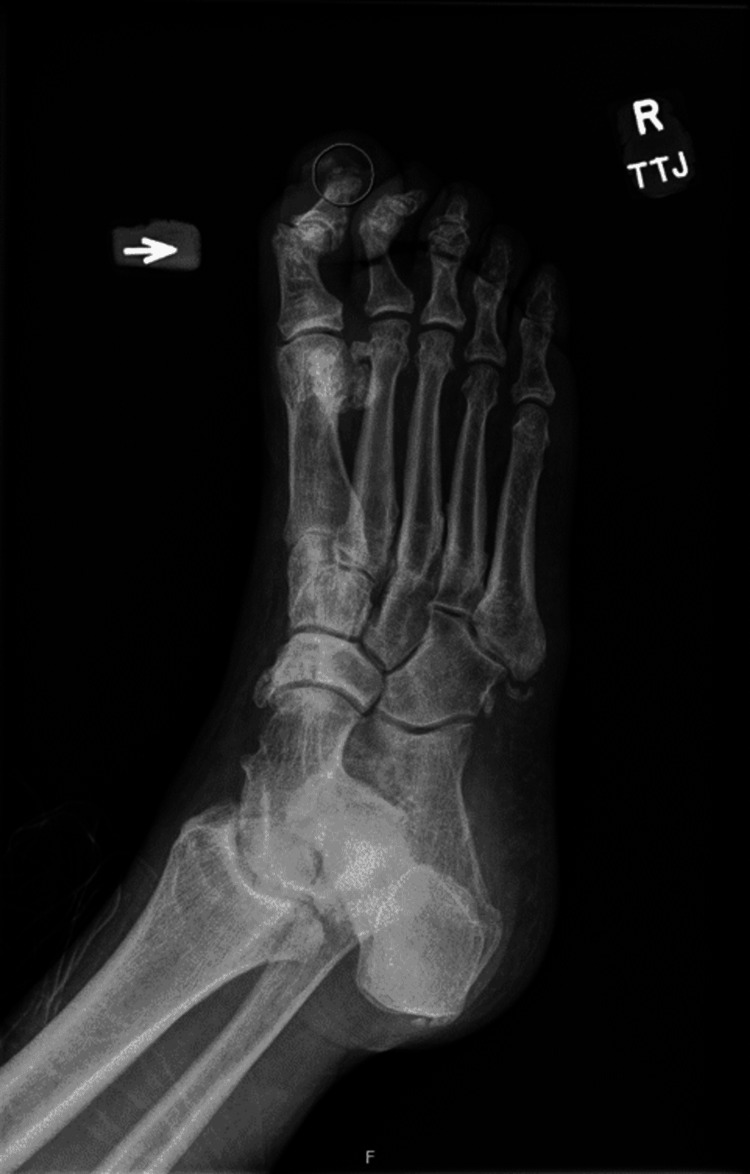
X-ray of right foot demonstrating soft tissue ulceration of the first digit with fragmentation at the tip of the first distal phalanx, concerning for acute osteomyelitis
